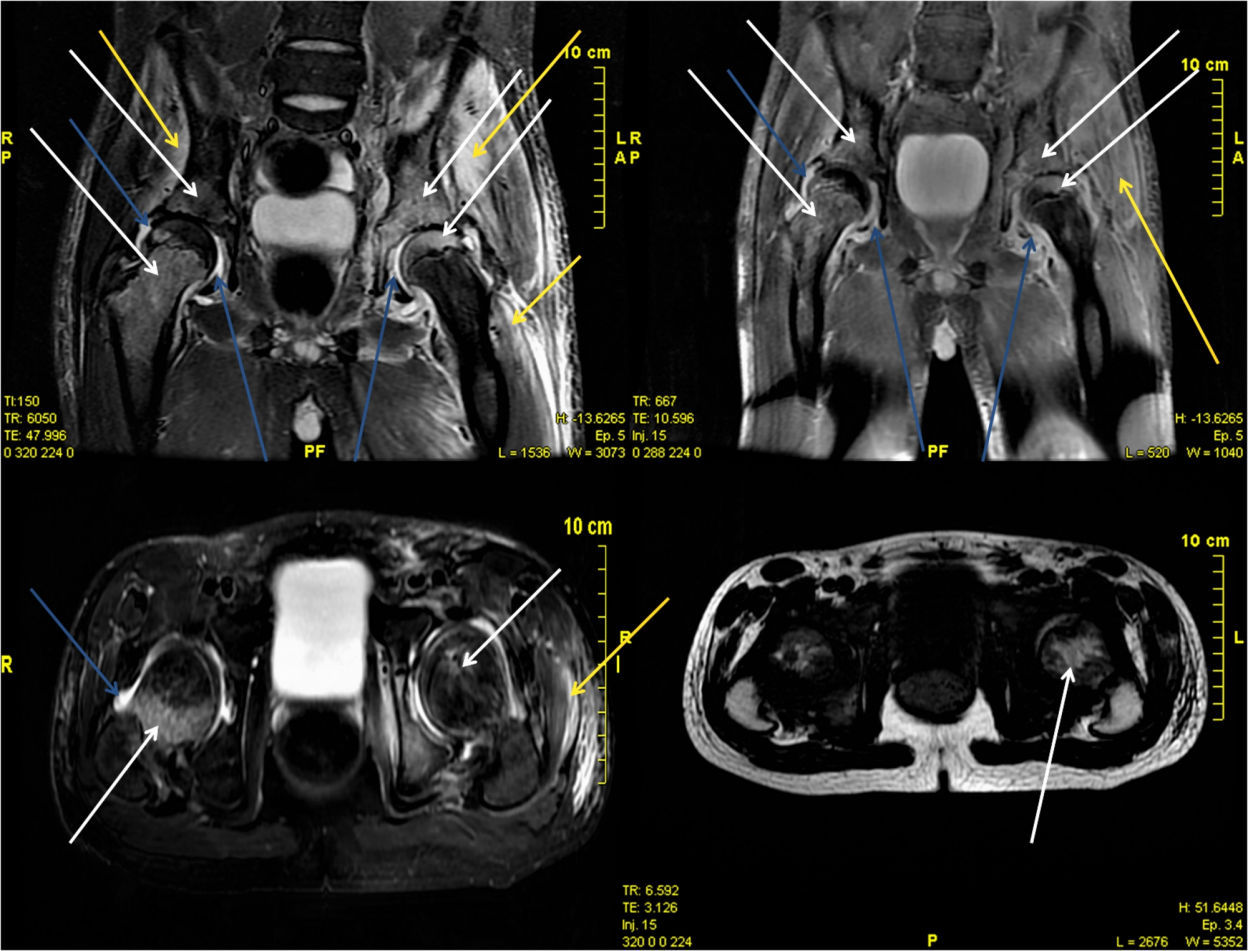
Magnetic resonance imaging (MRI) scan of patient No. 2. The white arrows point to bone involvement (osteomyelitis). The yellow arrows point to the soft tissue involvement (myositis). The blue arrows point to the arthrosis involvement (septic arthritis).
